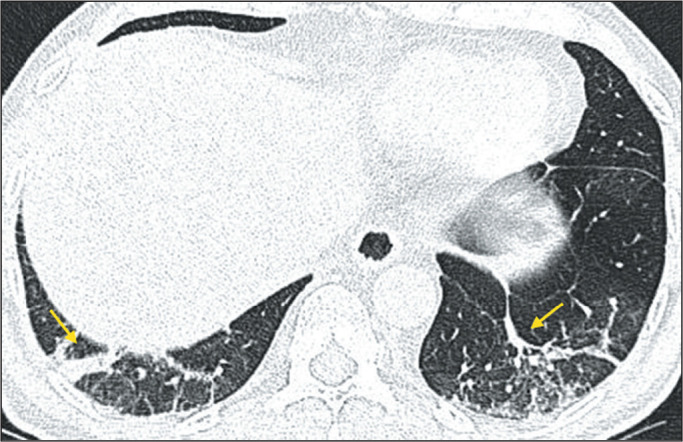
CT scan of a 54-year-old male patient, in a later disease stage, showing
